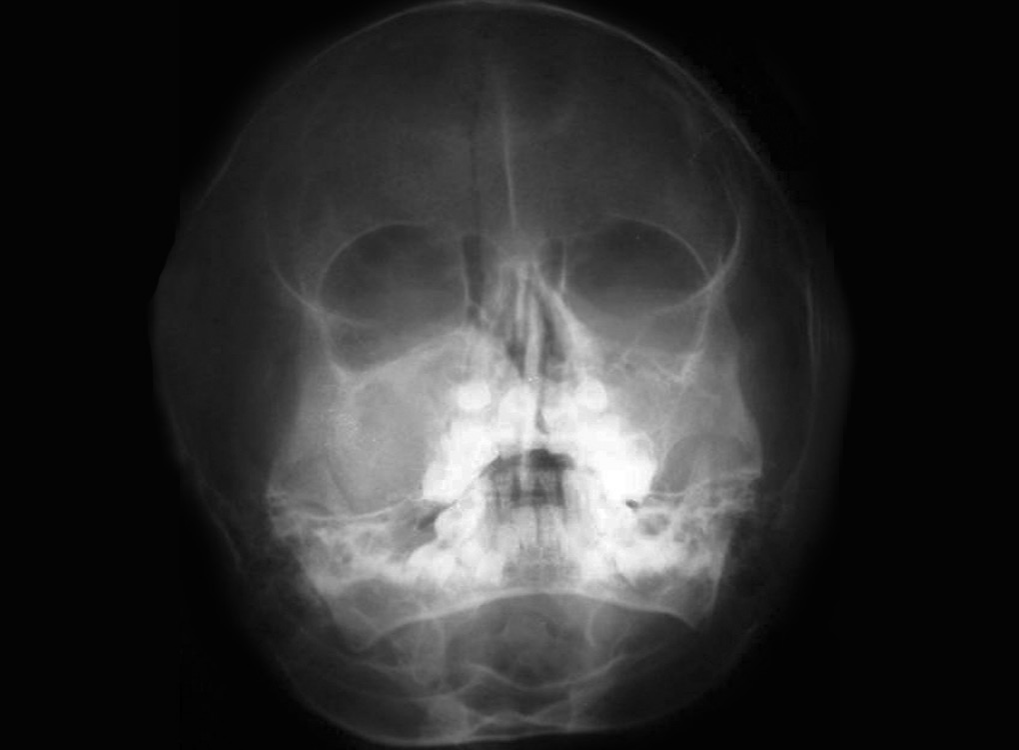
Extraoral radiograph reveals the presence of a lytic lesion of maxillary sinus with thinning out of zygoma
